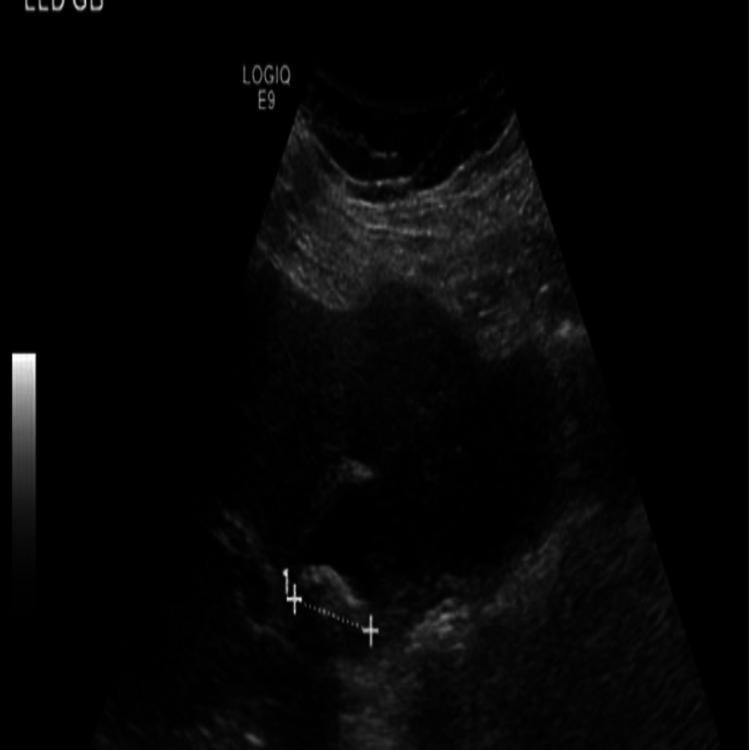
Ultrasound of the abdomen showing enlarged gallbladder, with thickened irregular wall likely inflammatory or malignant.
